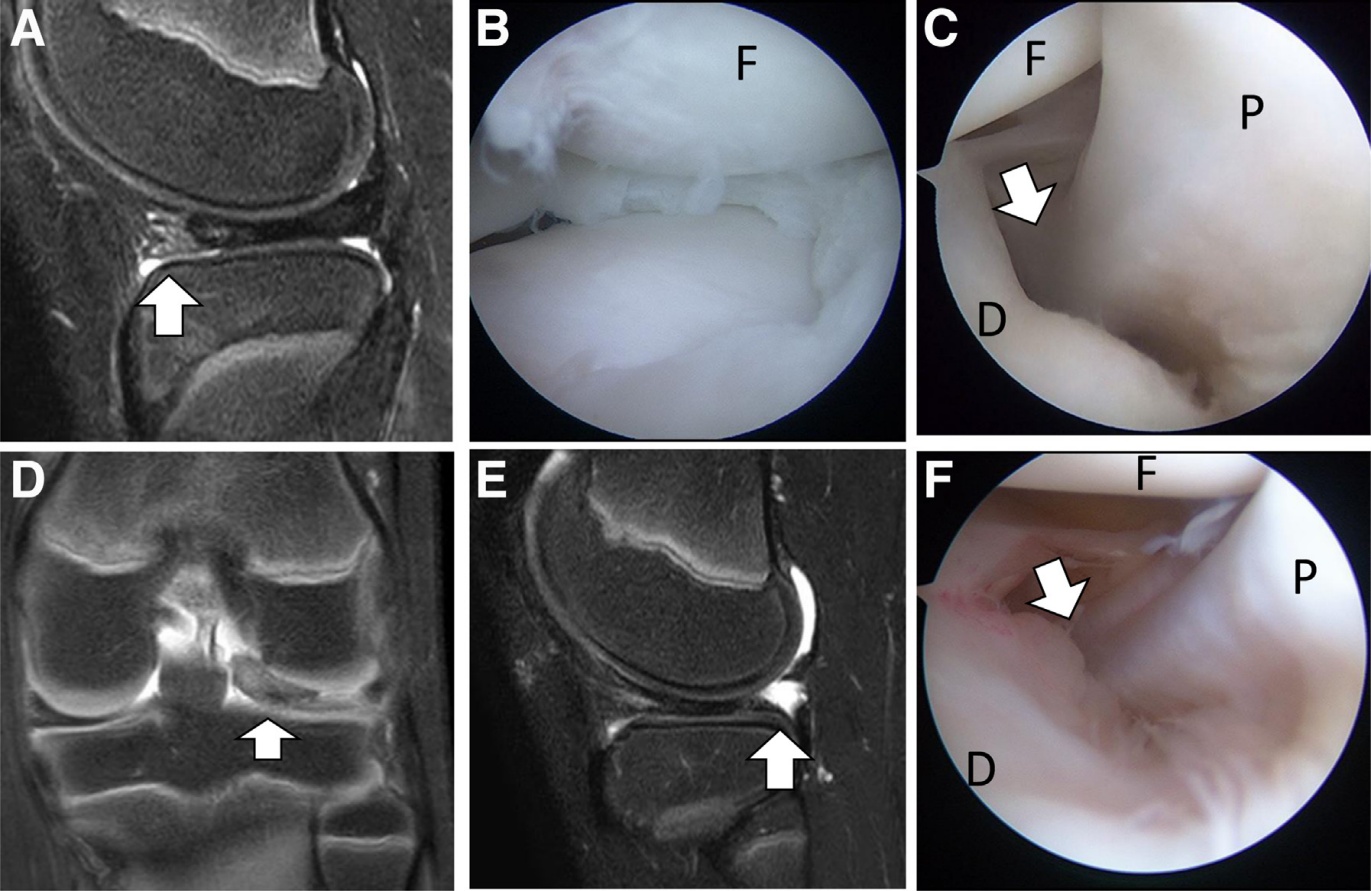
Newly developed case of posterior instability after anterior suture only for anterior instability of DLM in the left knee. (A) Anterior parameniscal soft-tissue edema (white arrow) was seen on the sagittal MRI. (B) Arthroscopic view after saucerization with transportal transcapsular repair for anterior instability from the anterolateral portal. (C) No hiatus widening (white arrow) was seen during first surgery. (D) The DLM is displaced anterocentrally (white arrow) on the coronal image after first surgery. (E) Meniscal loss of the posterior horn around the hiatus (white arrow) was seen on the sagittal images after first surgery. (F) Hiatus widening (white arrow) was seen in the revision surgery from the anterolateral gutter view. (D, discoid lateral meniscus; F, femoral condyle; MRI, magnetic resonance imaging; P, popliteal tendon.)
